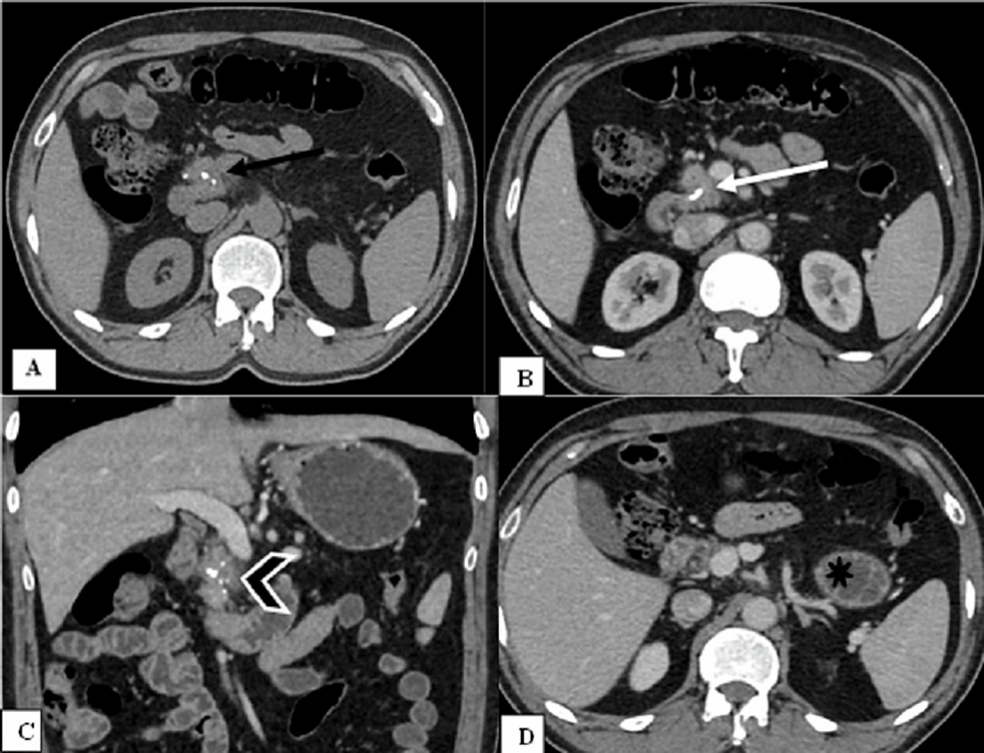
Agenesis of the dorsal pancreas with chronic calcific pancreatitis. Non-contrast computed tomography (A, black arrow) and contrast-enhanced computed tomography (B, white arrow) axial and coronal (C, chevron arrow) images of the abdomen show a slightly atrophic head of the pancreas with specks of calcification and non-visualization of the body and the tail of the pancreas. The dependent intestine sign (black star) can be seen in the subsequent image (D).
